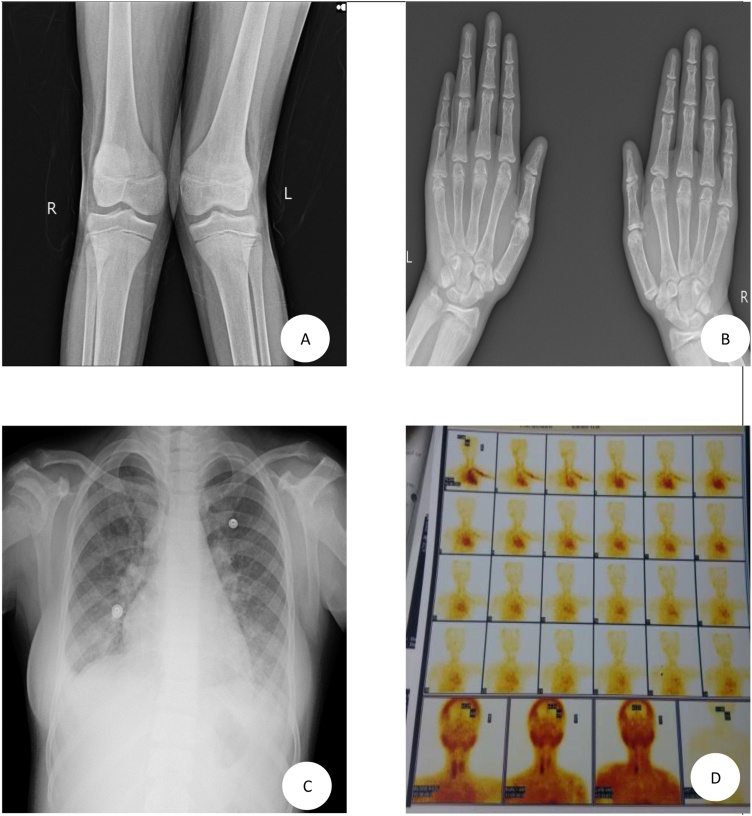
A: Radiograph bilateral both knee suggestive of genu valgum. B: radiograph bilateral both hand suggestive of acro-osteolysis. C: radiograph chest PA view suggestive of subchondral resorption on the distal ends of the clavicle. D: Tc 99 m Sestamibi scan suggestive of right inferior parathyroid adenoma.
